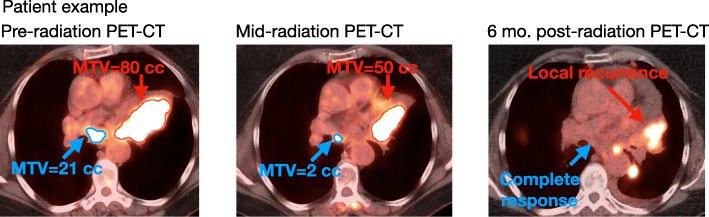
Representative patient example demonstrates per-lesion method of measuring MTV on fused PET-CT images. As shown, a nodal lesion had a complete response to chemoradiation while the primary tumor had large residual MTV at mid-radiation PET-CT with subsequent local recurrence
